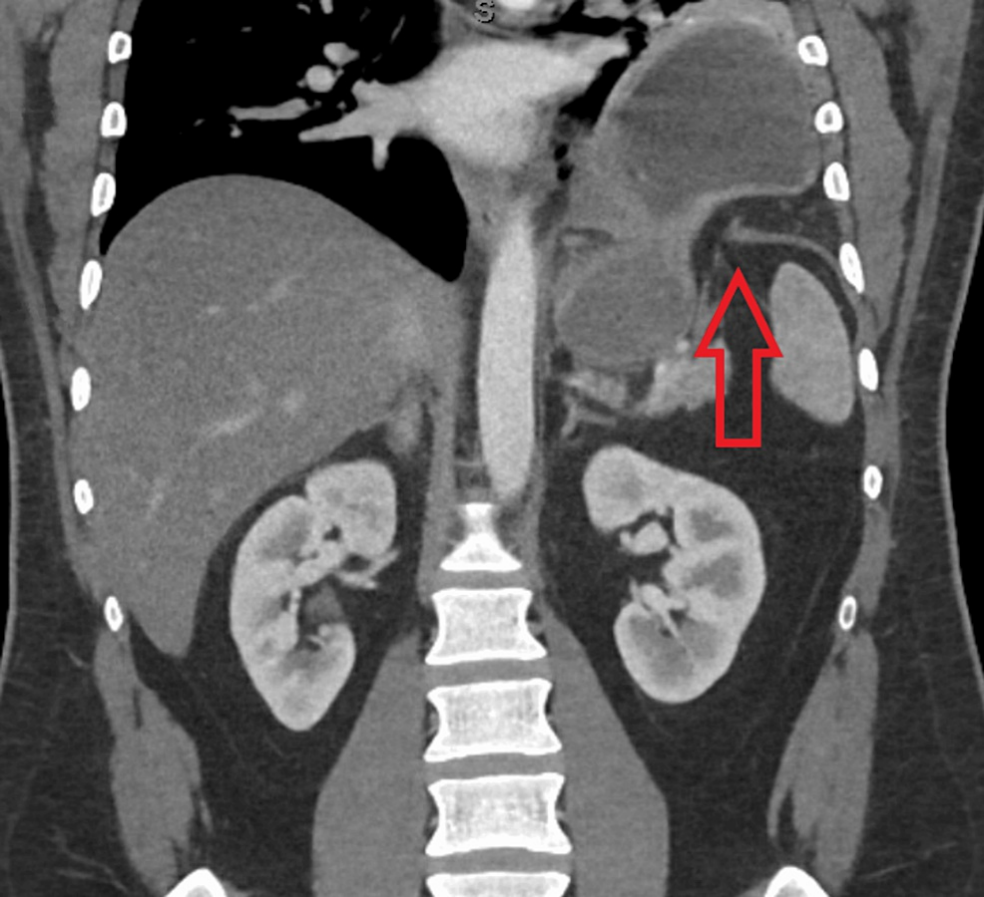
Coronal slice of CT scan two months post-injury, showing left diaphragmatic hernia with partially intrathoracic stomach. CT - Computed tomography
